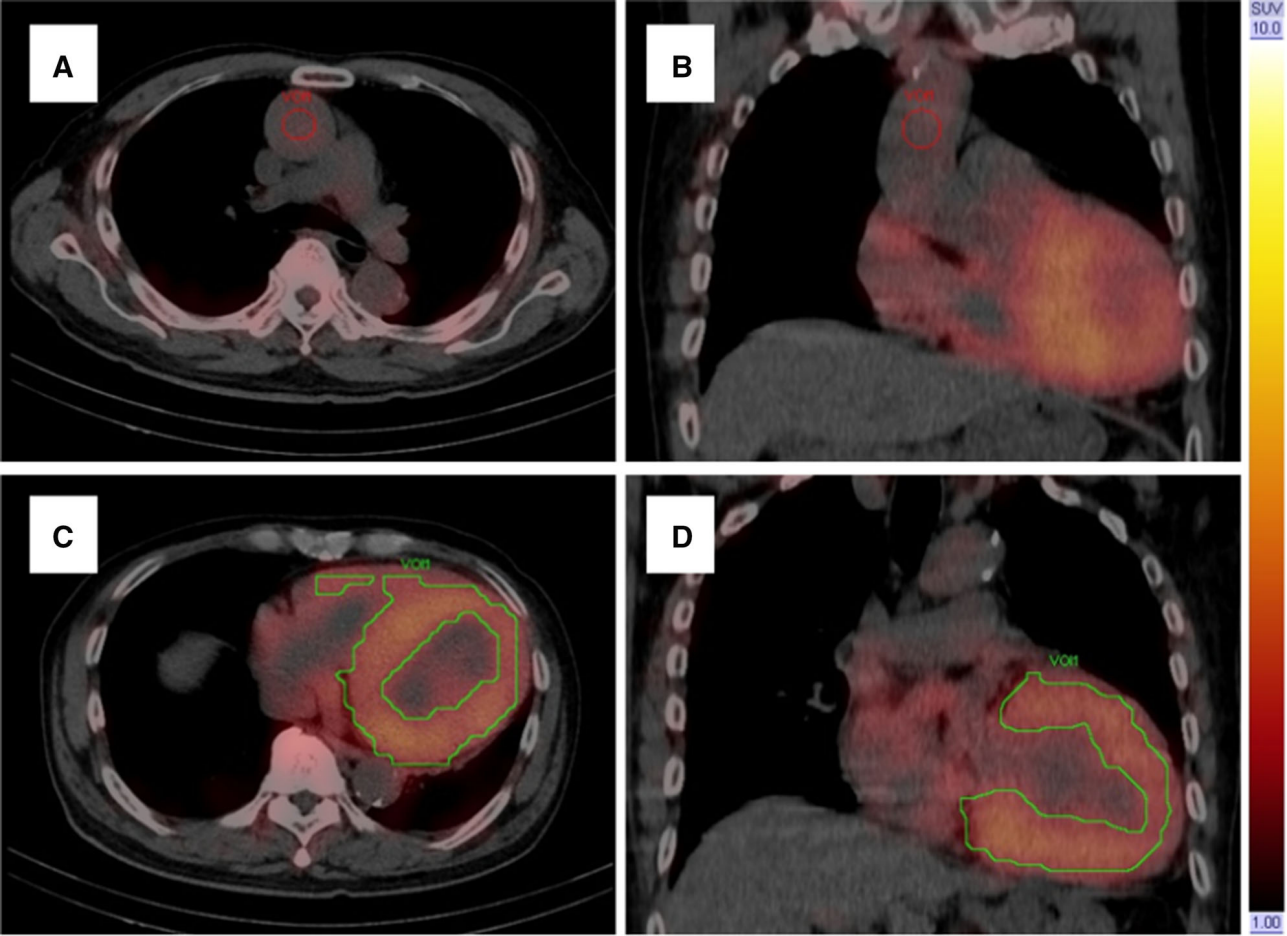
An example of SUV-threshold volumetric evaluation of ^99m^Tc-PYP SPECT/CT at 1 h after radiotracer injection. (A, B) Red circles is spherical volume of interest placed in center of ascending aortic blood pool. (C, D) Green contours indicate myocardial regions with ^99m^Tc-PYP uptake > 1.4 × aortic blood pool radioactivity (SUVmax) automatically evaluated using xSPECT Quant. ^*99m*^*Tc-PYP*, Technetium-99m pyrophosphate; *SPECT*, single-photon emission computed tomography; *SUV*, standardized uptake value
